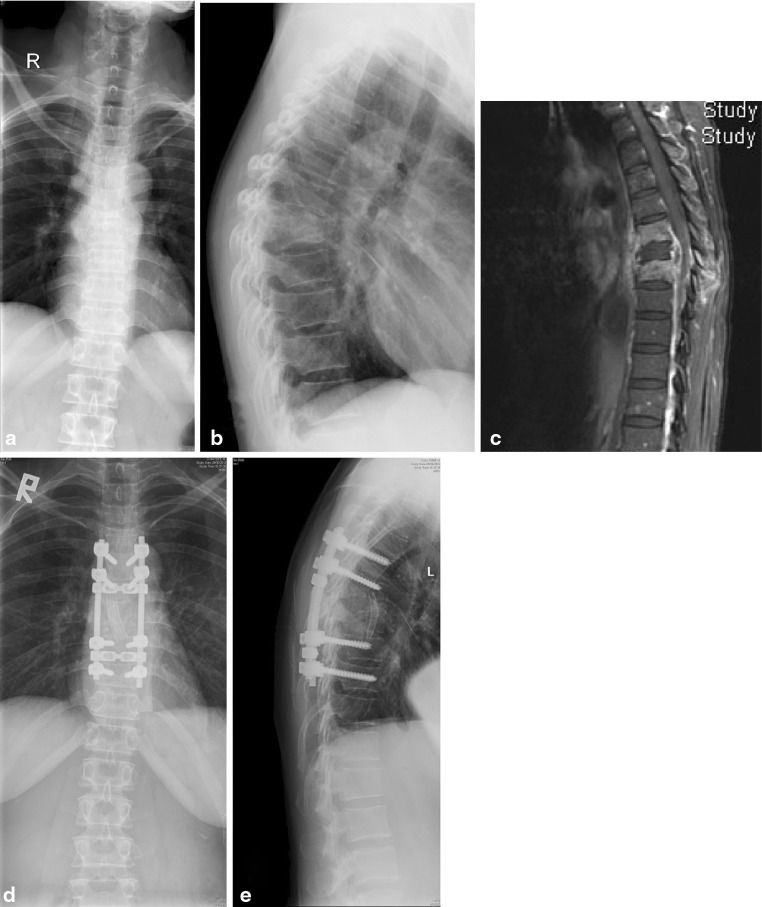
A case requiring anterior debridement, and rib strut grafting (the “Hong Kong Operation”) combined with posterior instrumentation to preserve the sagittal alignment in a case with multi-level infection. Pre-operative X-rays (a, b) and the MRI (c) showing the extent of involvement, and post-operative X-rays (d, e) showing restoration of normal kyphosis
